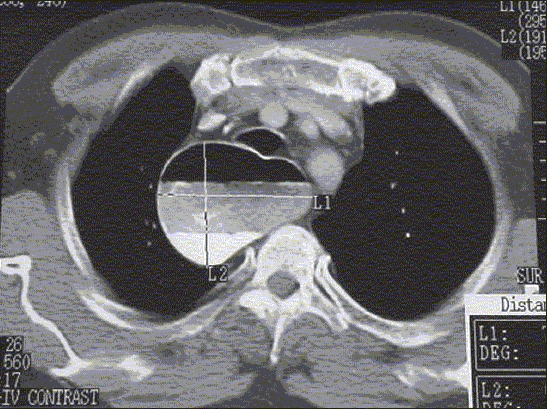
CT scan chest and abdomen
